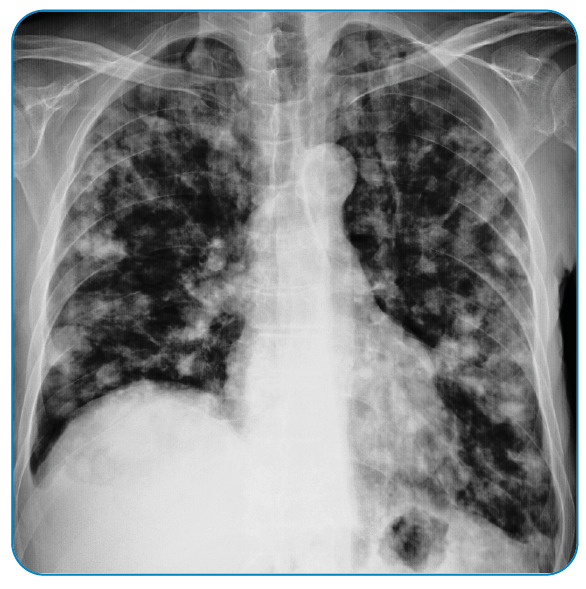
Chest radiograph shows multiple metastatic nodules in all lobes of both lungs.
